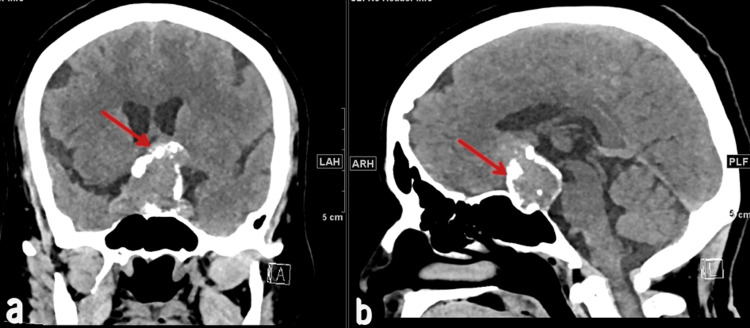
A non-contrast-enhanced brain CT scan showing a pituitary mass with curvilinear peripheral thick calcification in coronal (a) and sagittal (b) views, indicated by red arrows
